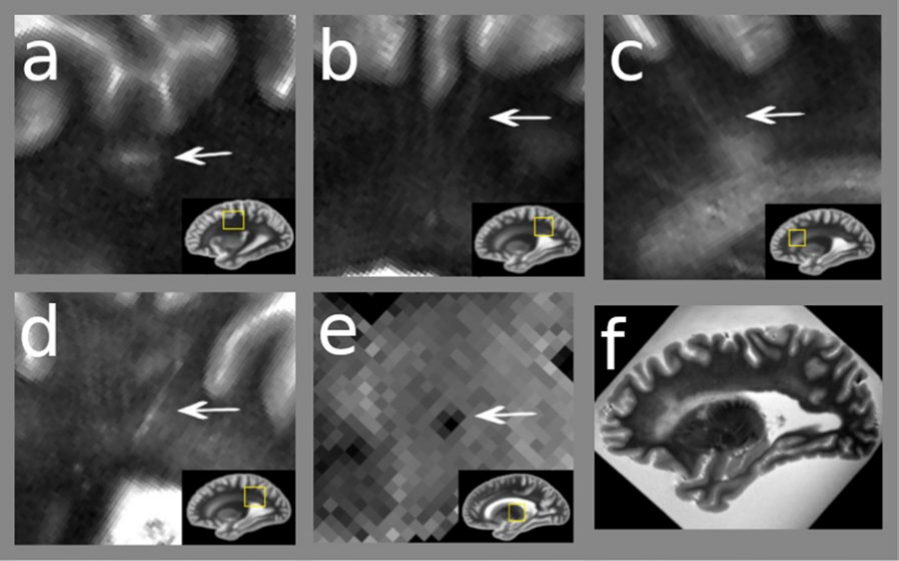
MRI brain findings. a MRI image from case 13 shows an acute infarct. b EPVS in left hemisphere. c and d, EPVS in right hemisphere, e Microbleed in basal ganglia. f White matter hyperintensities in overall brain
